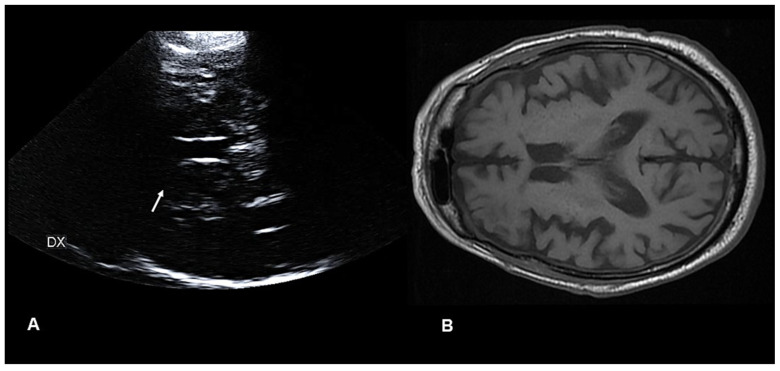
(A) (*left panel*) Parenchymal transcranial sonography showing the complete resolution of the hyperechogenicity in the right (DX) lenticular nucleus previously observed (*white arrow*); (B) (*right panel*) axial brain magnetic resonance imaging showing normal T1-weighted intensity in the right putamen.
